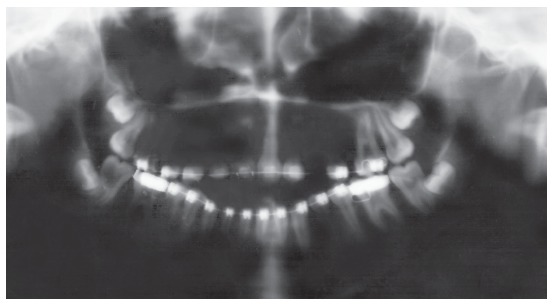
Panoramic radiograph with the fixed appliances.
